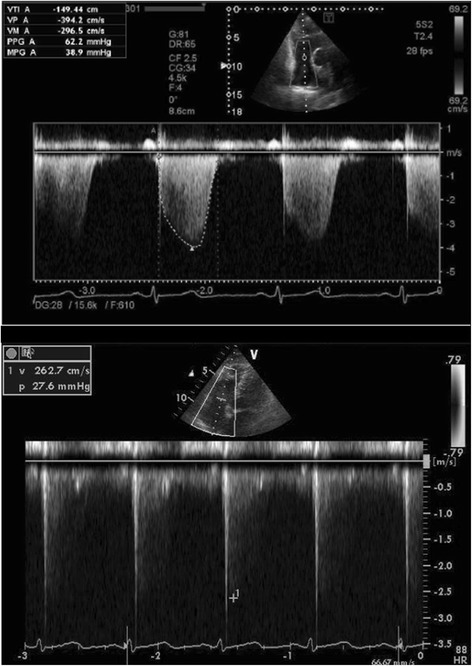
Two-dimensional Doppler echocardiographic images of tricuspid regurgitation 1 month before LDLT (upper) and 2 months after LDLT (bottom)
